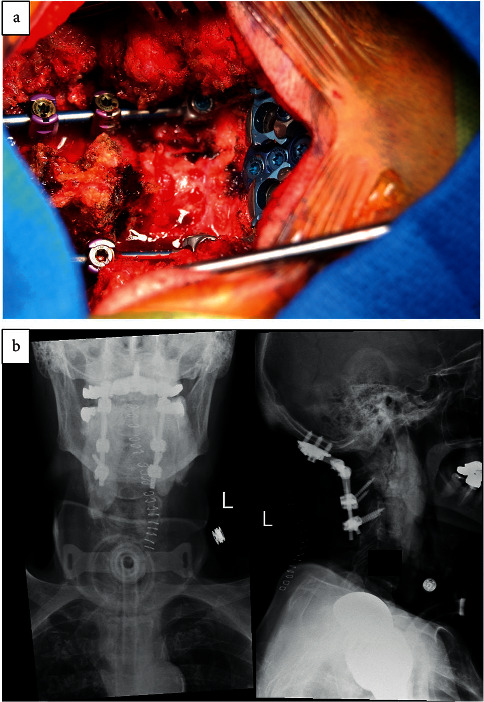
(a) Intraoperative photo of the posterior occipitocervical (occiput to C3) fusion with a posterior C1 laminectomy. (b) Postoperative AP and lateral x-rays of the cervical spine showing the construct.
